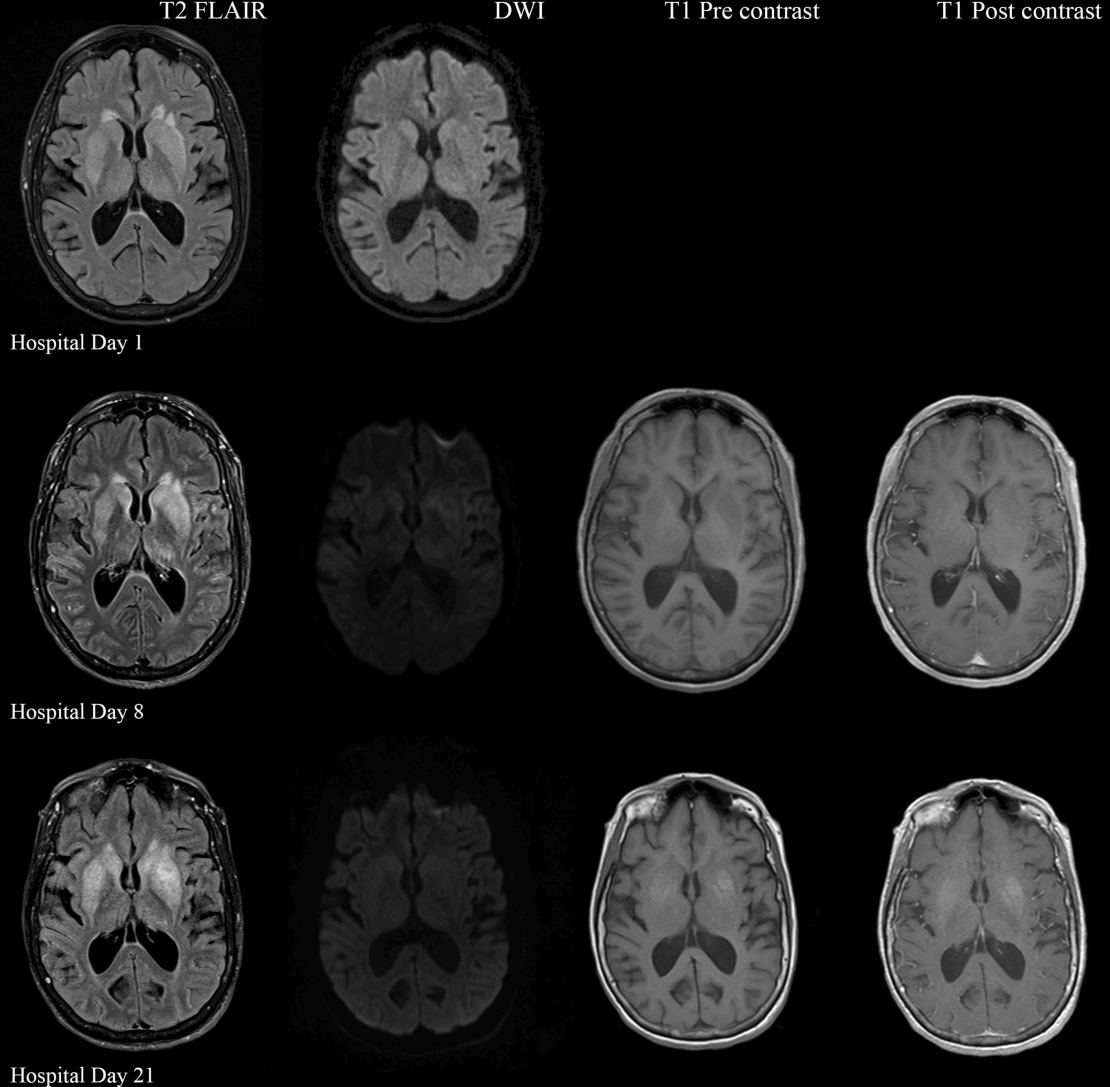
Serial MRI Brain sequences for hospital day 1, 8, and 21. On hospital day 1, there is abnormal hyperintense T2 FLAIR signal in the bilateral deep gray nuclei without associated restricted diffusion. On hospital day 8, there is progressively increasing abnormal T2 signal in the deep gray nuclei as well as restricted diffusion within the areas of abnormal T2 signal. There are no abnormalities on T1 pre and post contrast sequences. On hospital day 21, there is evolving T2 hyperintense signal in the bilateral deep gray nuclei with similar degree of restricted diffusion. There is now an abnormal T1 hyperintense signal in the deep gray nuclei without contrast enhancement, suggestive of developing gliosis.
